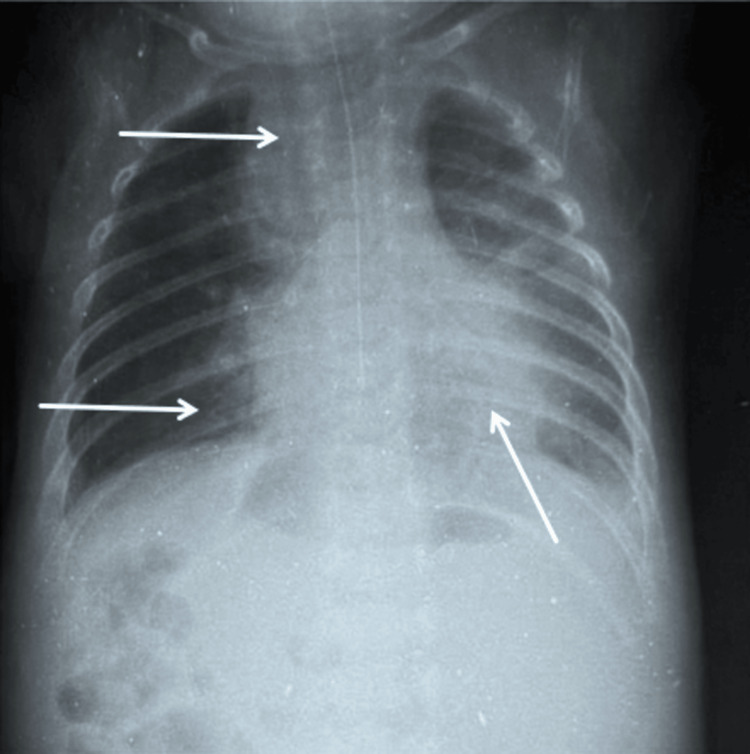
Chest radiograph (day 7) showing progression to pulmonary opacities
